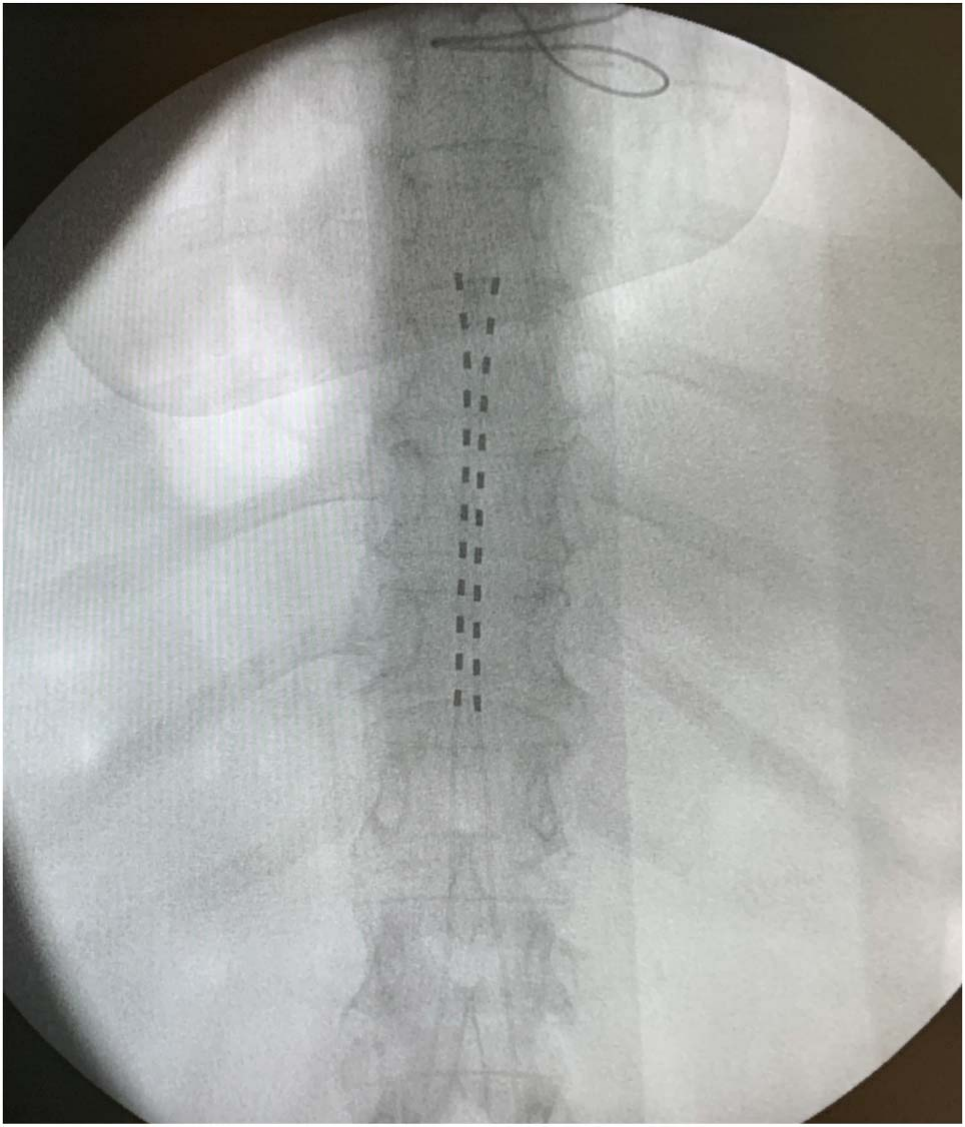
Anteroposterior radiographic view of the implanted closed-loop SCS in the patient, consisting of two 12-pole electrodes, with the upper electrode positioned at the lower endplate of T8. SCS, spinal cord stimulation.
